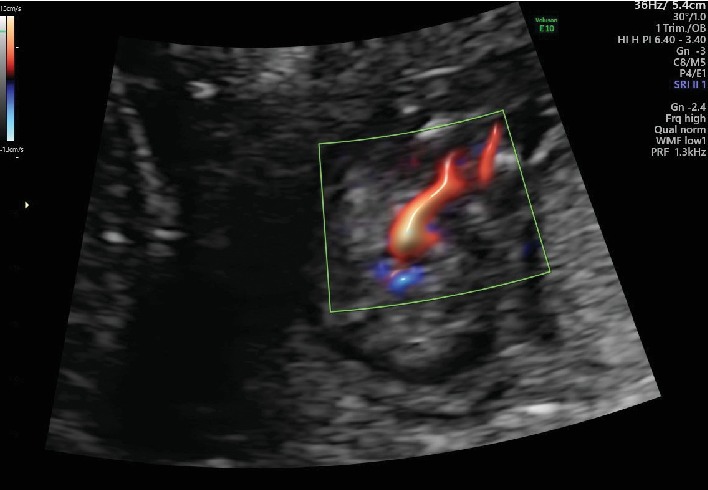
Color Doppler ultrasonography at the 13 weeks of gestation. A single umbilical artery was seen.
